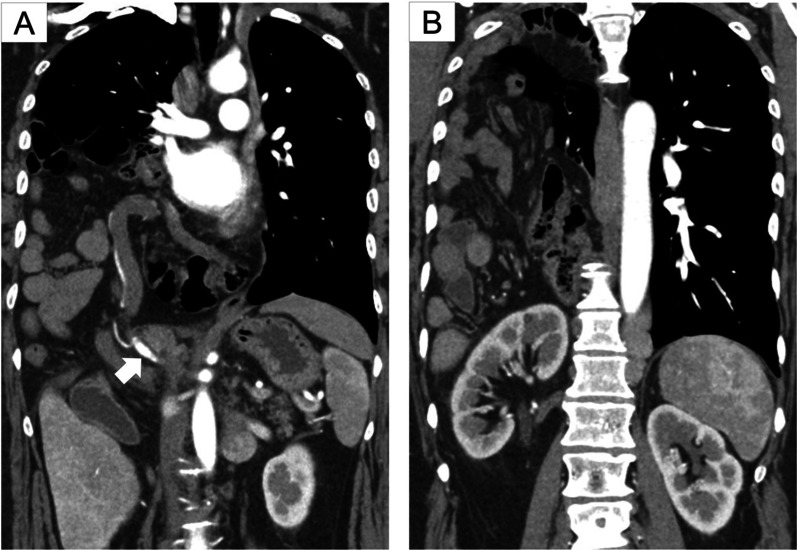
Coronal views of preoperative contrast medium-enhanced thoracoabdominal computed tomographic scans. a Small intestine, the colon, and the main trunk of the superior mesenteric artery (white arrow) had herniated through the diaphragmatic defect into the right thoracic cavity. b Right kidney was detached from the retroperitoneum and had also herniated into the right thoracic cavity
